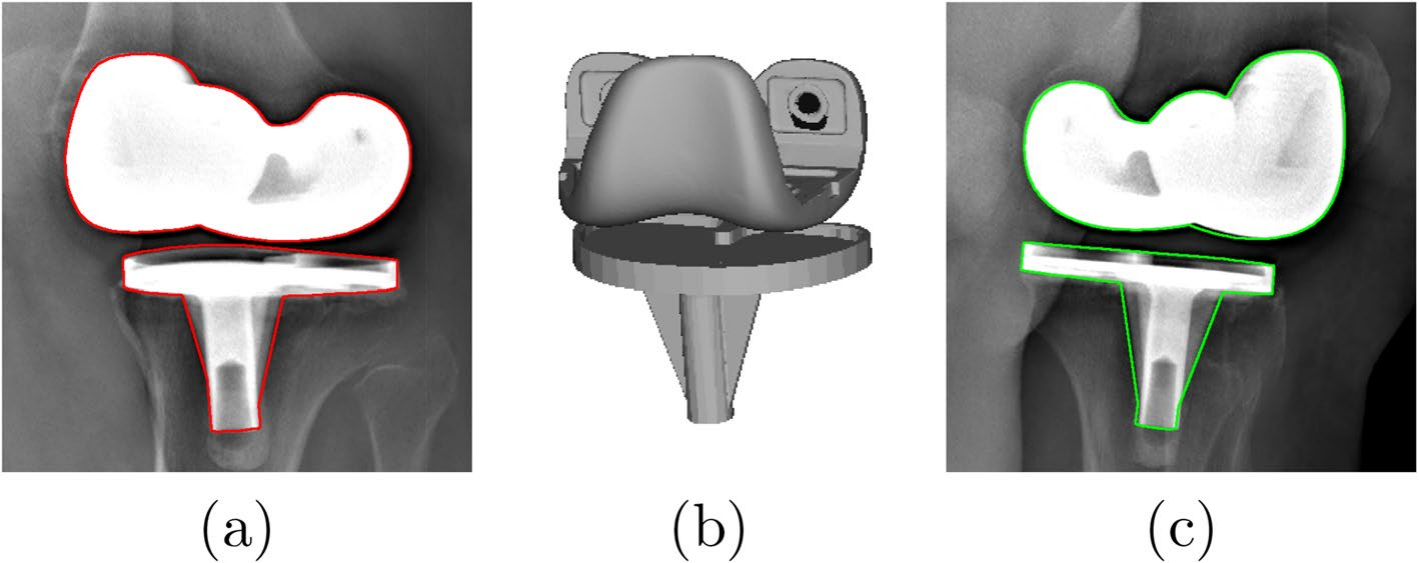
Example of 3D/2D registration result on real radiographic images. a, c component external contours projected on 45-degree image and 135-degree image, and (b) 3D view
